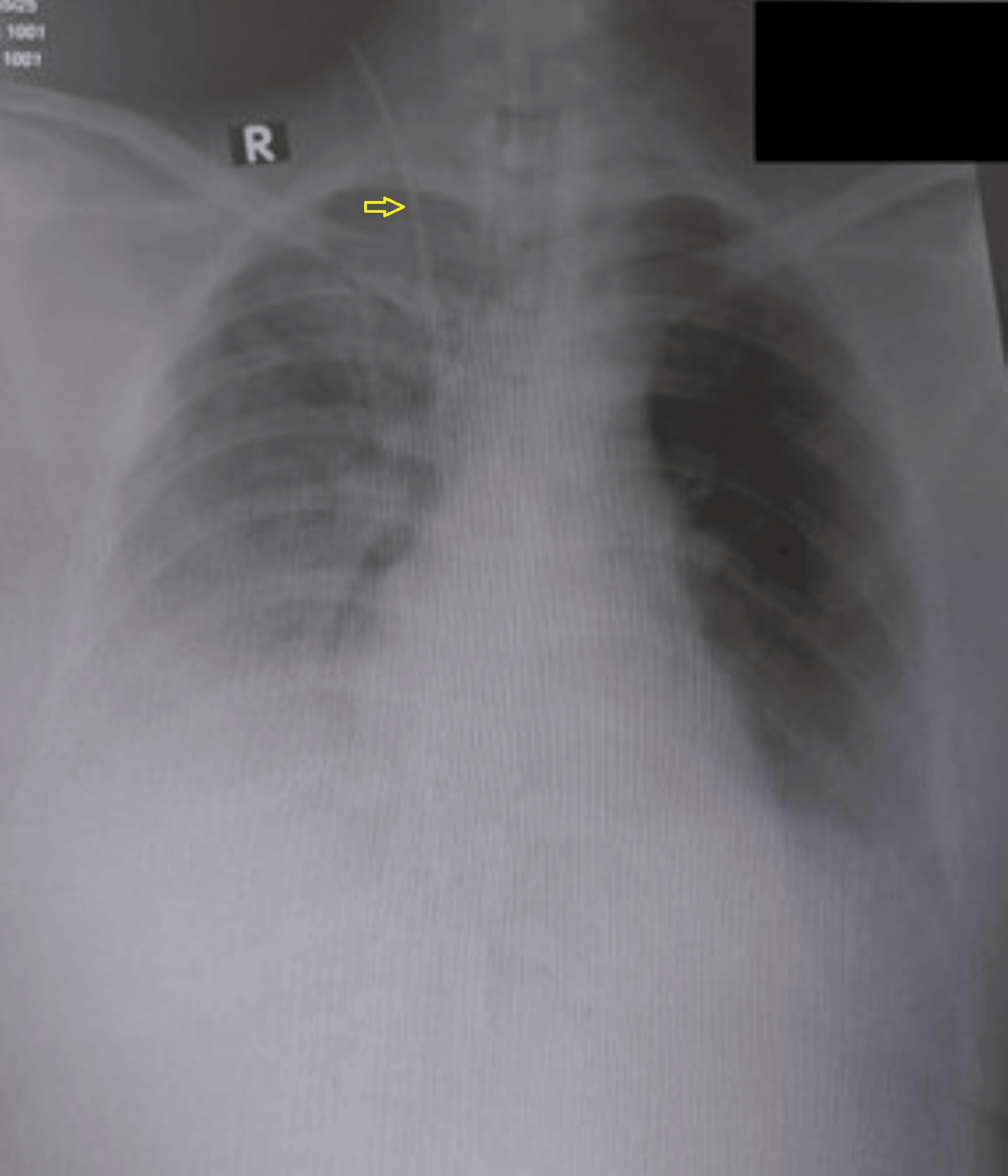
Chest X-ray (anteroposterior view) showing bilateral diffuse haziness, more prominent in the lower zones, with blurred lung markings. The right internal jugular vein central catheter is in situ (arrow).
